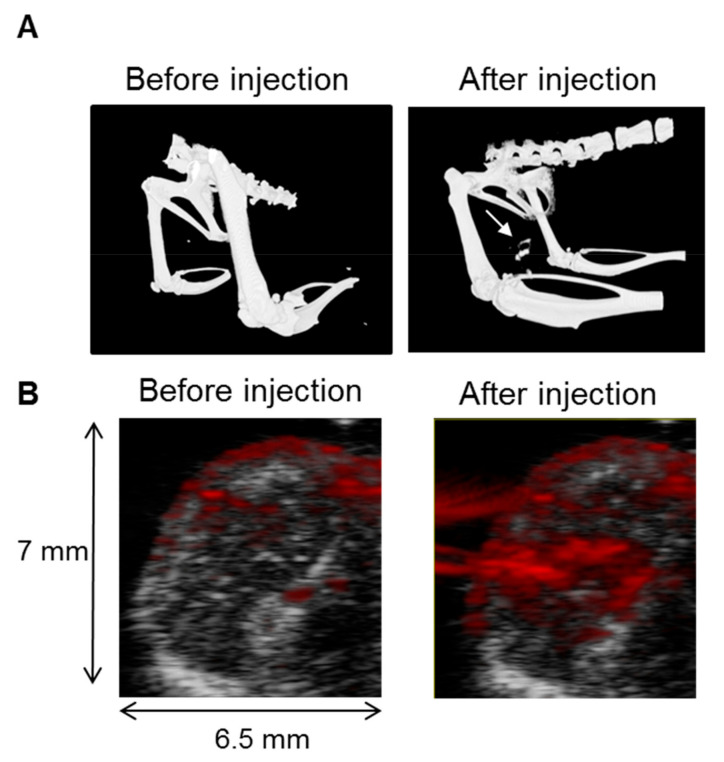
(A) CT images before and after injection of gold nanostars into the tumor. An increased contrast was noticed at the tumor site after gold nanostars injection as indicated by the arrow; (B) In vivo PA images before and 24 h after nanostar injection. The photoacoustic imaging (PAI) signal (red pixels) is overlaid over the anatomical ultrasound images (grey pixels).
